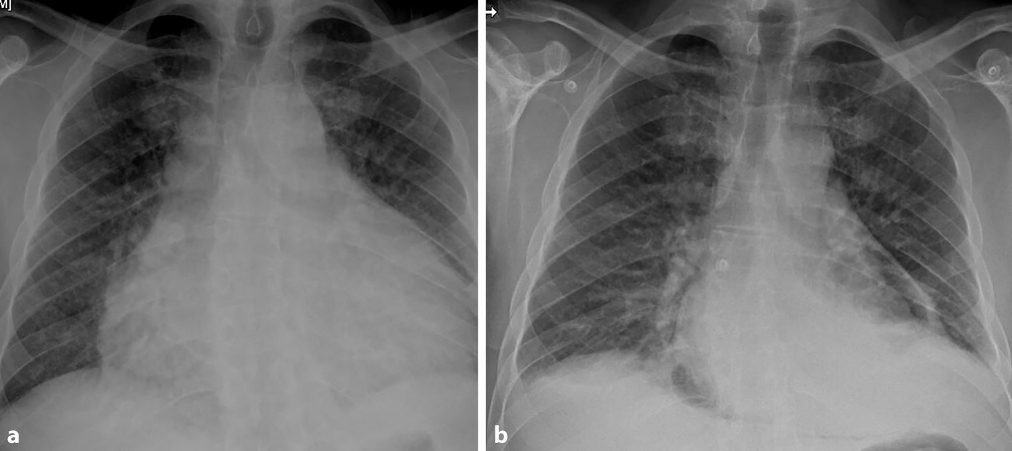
Chest radiographs, a obtained at admission showing enlarged cardiac silhouette due to pericardial effusion and b obtained 24 h after pericardiocentesis showing iatrogenic pneumopericardium
